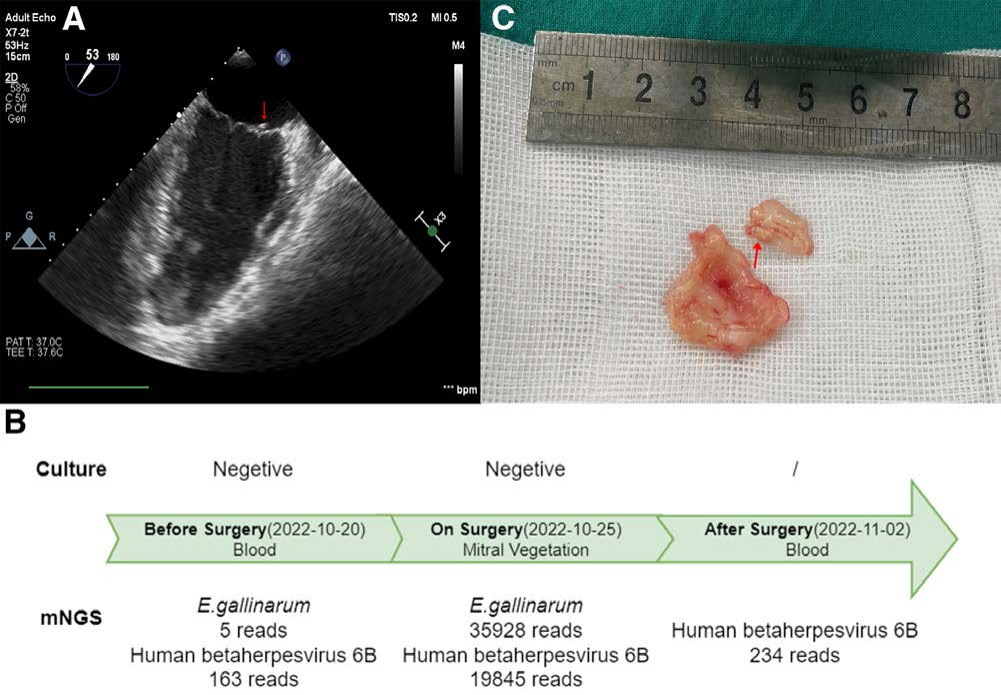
The diagnosis of infection. (A) Cardiac ultrasound. Arrows indicate the observed endocardial vegetations. (B) Pathogenic microorganisms detected by culture mNGS before and on surgery. (C) Heart valve vegetation obtained during surgery. mNGS = metagenomic next-generation sequencing.
